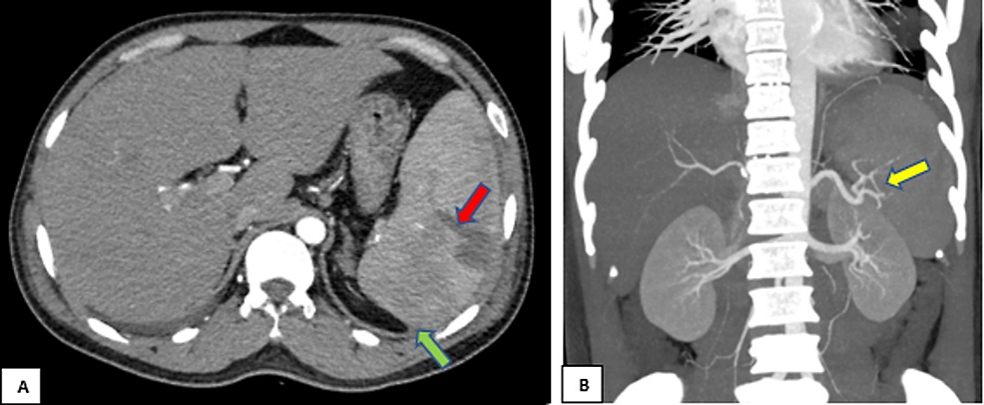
Computed tomography with intravenous contrast A) axial and B) coronal cuts showing a hypodense parenchymal laceration at the posterior aspect of the spleen (red arrow) with mild perisplenic hematoma (green arrow) and intact splenic artery (yellow arrow)
